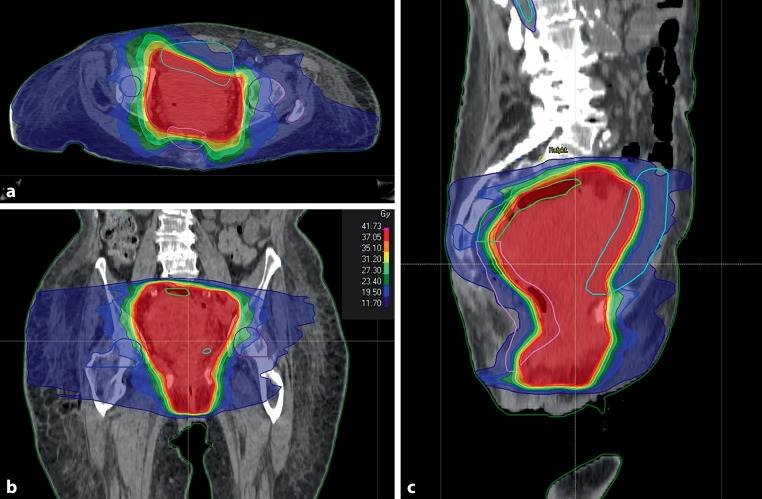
An 83-year-old woman with palliative hemostatic RT for endometrial cancer receiving 6‑MV photon intensity-modulated radiotherapy with 39 Gy in 13 fractions for a planning target volume of 1634 cm^3^ resulting in cessation of bleeding after 12 days (equivalent dose in 2‑Gy fractions for α/β of 10 = 24 Gy) (a: axial, b: coronar and c: sagittal computed tomography slices with the resulting radiotherapy plan)
